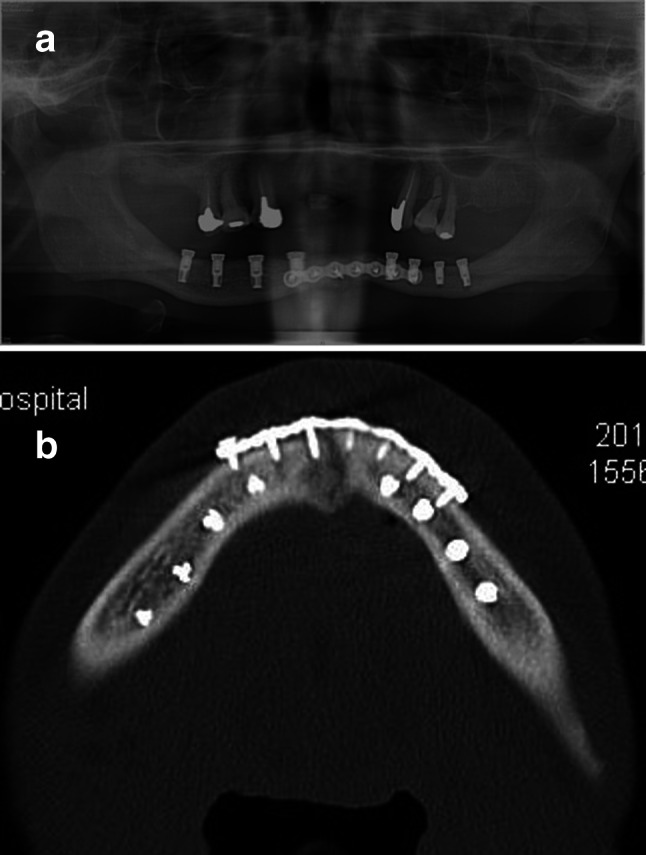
a Panoramic radiograph after implant bodies placement and b a fracture line is seen in the midline of the mandible on horizontal CT image. No contact between the implant bodies and the screws is allowed
